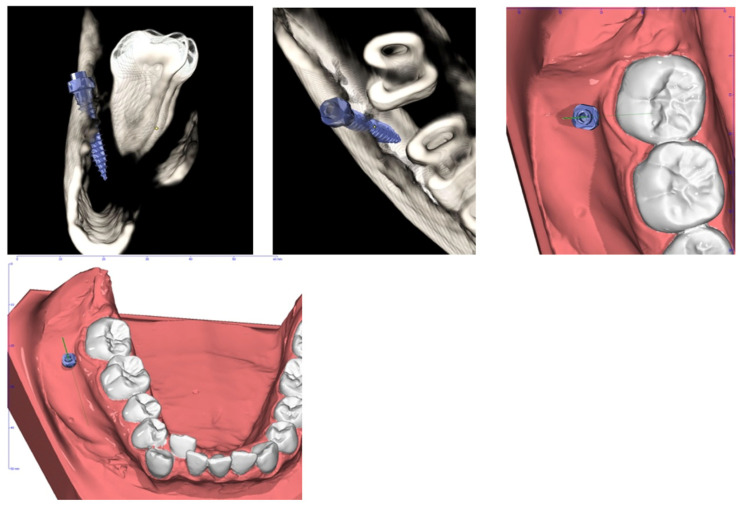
Planning of miniscrew insertion (Ortodontica Italia, Rome, Italy) on CBCT (Cone Beam Computed Tomography) from different views.
